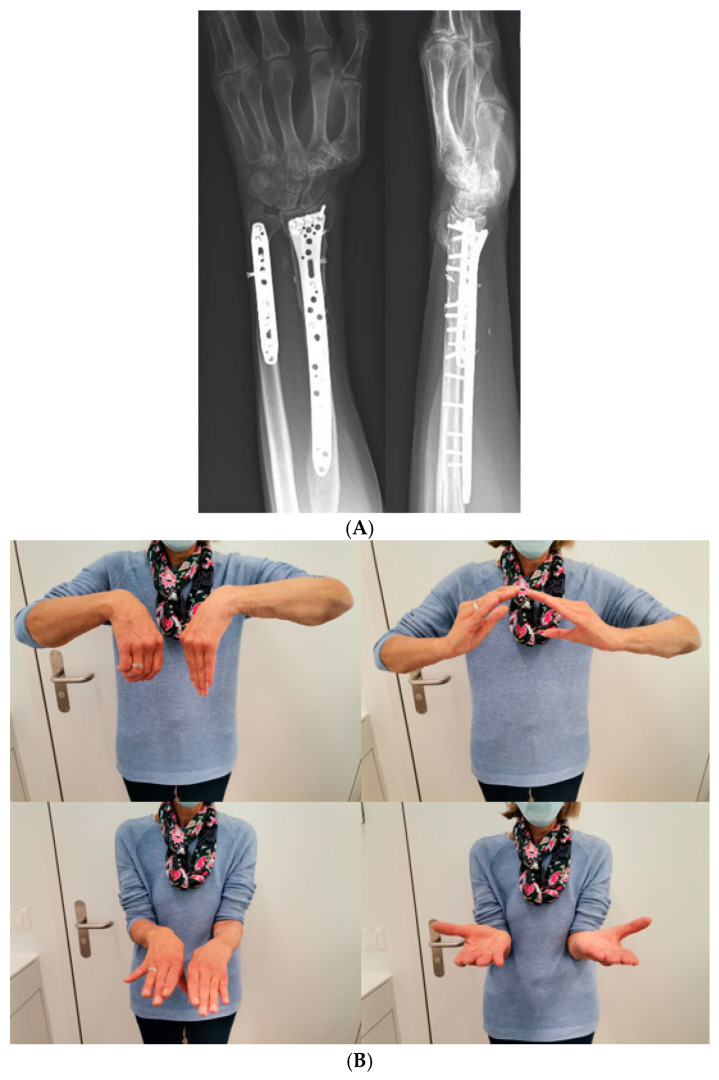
(A) X-ray in two planes at follow-up after 6 months, (B) clinical result after 6 months.
